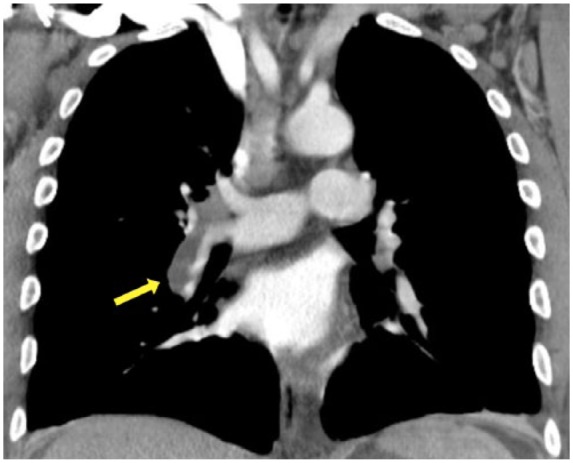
Computed tomography scan of chest with contrast. Large acute pulmonary embolus involving the right pulmonary artery extending into the interlobar artery and its branches (yellow arrow).
